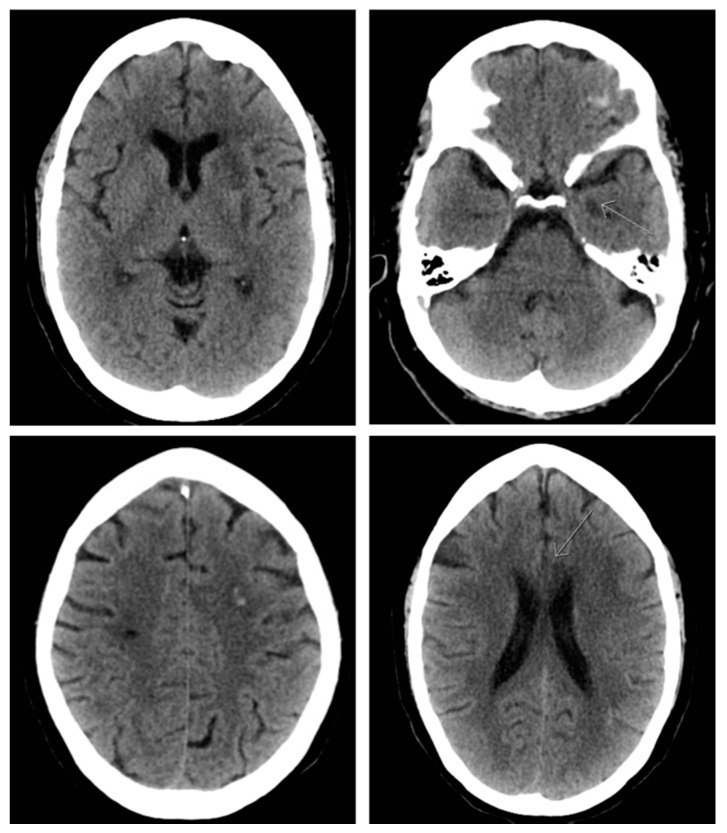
Non-contrast head CT showing multiple areas of small petechial hemorrhage 24 hours following the second mechanical thrombectomy.
